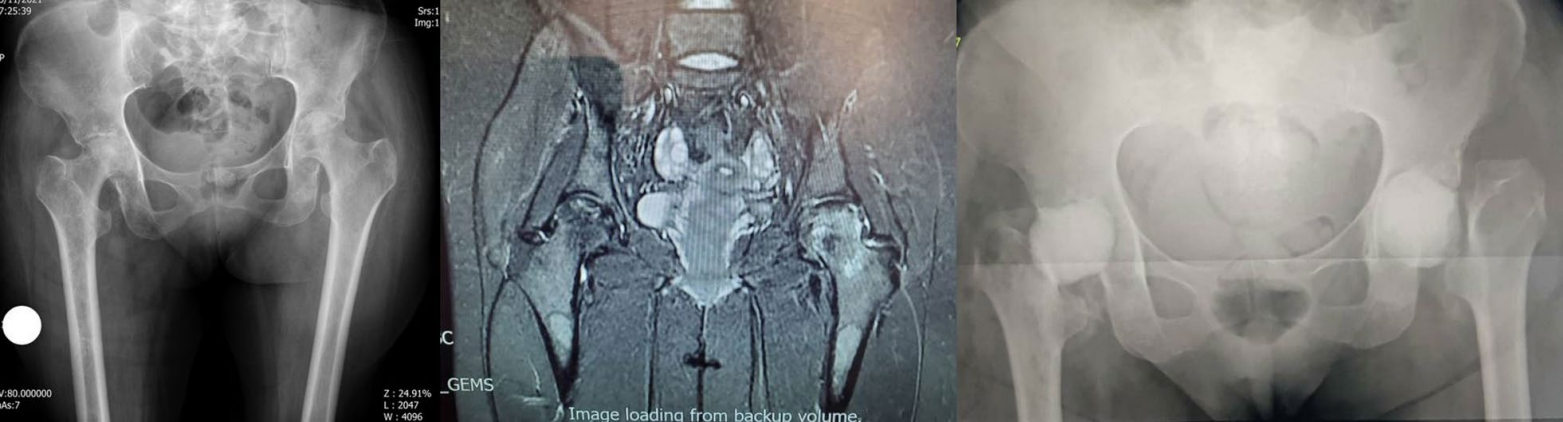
a Case four, preoperative X-ray, both sides involvement. b MRI of the hips showing bilateral joint destruction and cartilage loss. c Post-op radiography after first stage of THA for both sides
